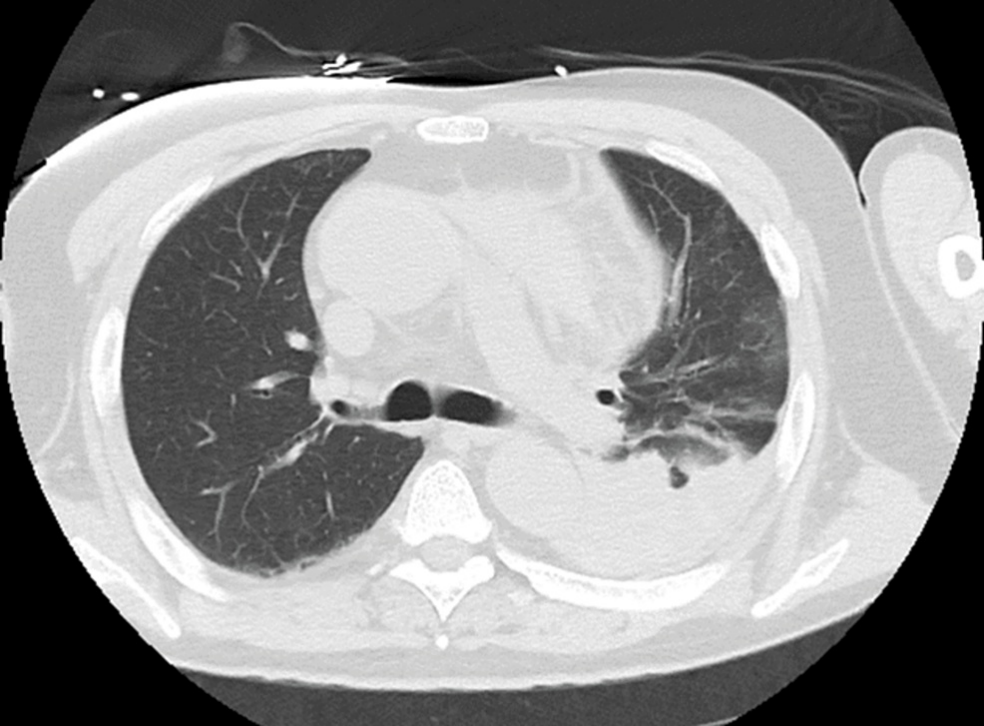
The lung field CT image of a COVID-19-positive patient (Case 2). There is no obvious infiltrative shadow in the lung field, but a hematogenous pleural effusion associated with rupture is observed in the left thoracic cavity.
